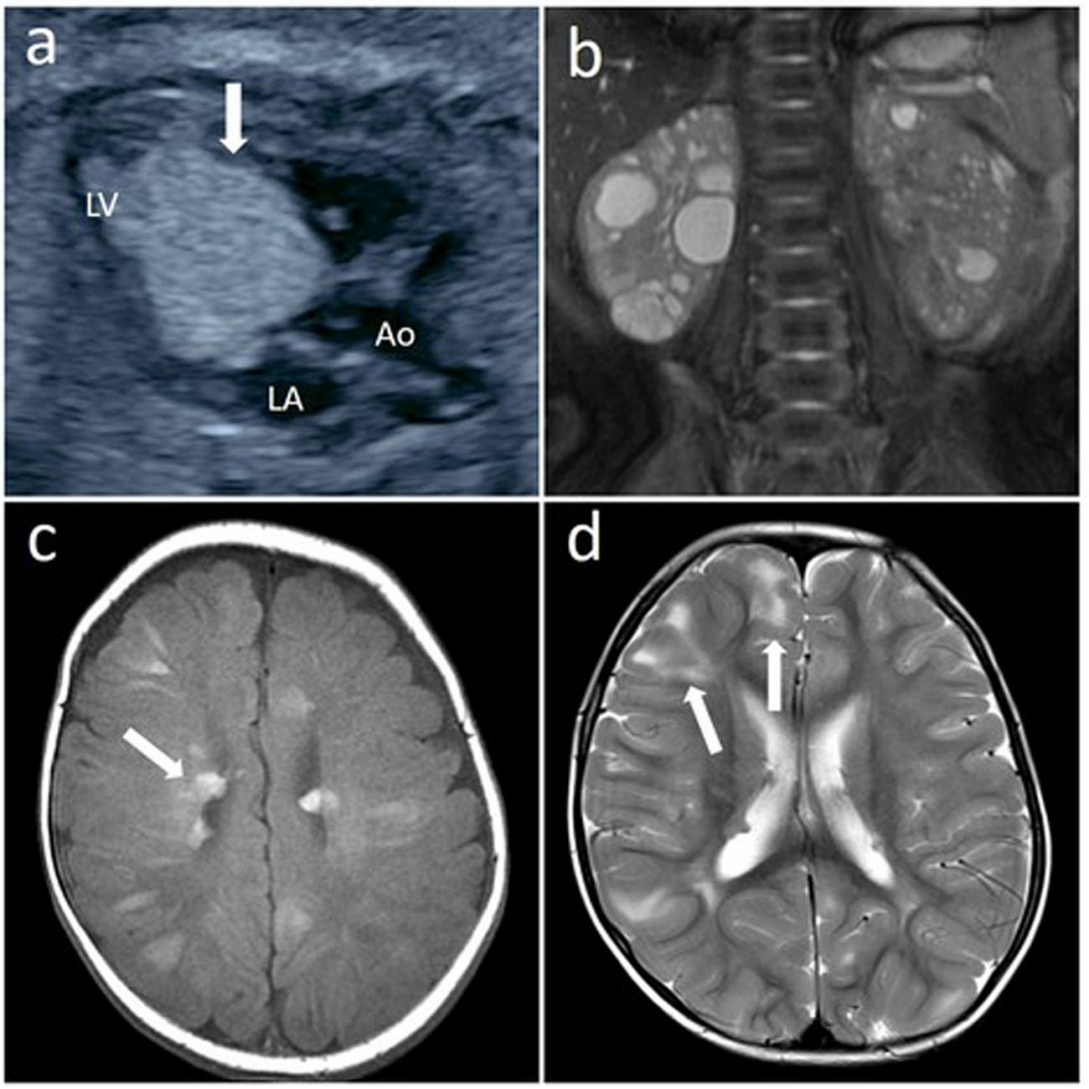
Representative image findings. a Fetal echocardiography showing rhabdomyoma (white arrows), Ao aorta, LA left atrium, LV left ventricle. b Magnetic resonance imaging (MRI, T2-weighted image) of abdomen at 3 months old showing enlarged kidneys with multiple cysts. c MRI (T1-weighted image) of brain at 55 days old showing multiple subependymal nodules along the lateral ventricle walls (white arrows). d MRI (T2-weighted image) of brain at 3 years old showing multiple subcortical high signal lesions indicating cortical tubes in cerebral hemisphere, predominantly in frontal lobe (white arrows).
